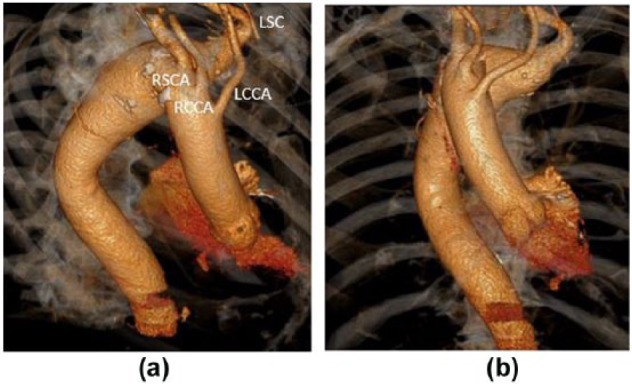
Pre-operative volume rendering CT angiogram imaging demonstrating: (a) a right-sided arch, aberrant origin of the left subclavian artery and (b) a Kommerel’s diverticulum at its origin. LSA: left subclavian artery; LCCA: left common carotid artery; RCCA: right common carotid artery; RSA: right subclavian artery.
